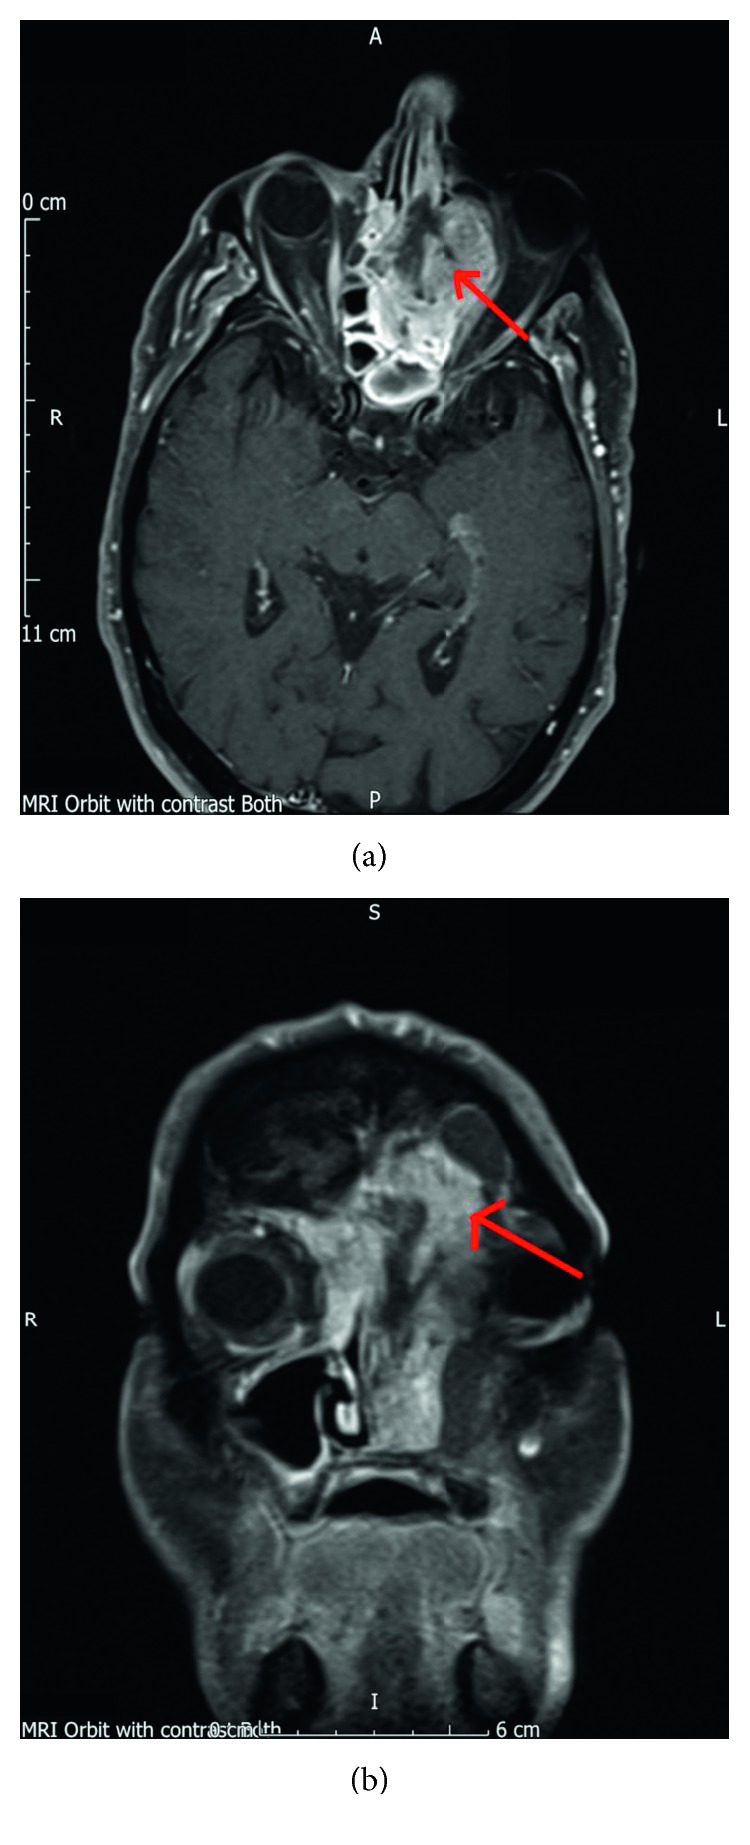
MRI head scan shows breaching of dura mater. Red arrow denotes lesion of interest. (a) Axial slide. (b) Coronal slide.
